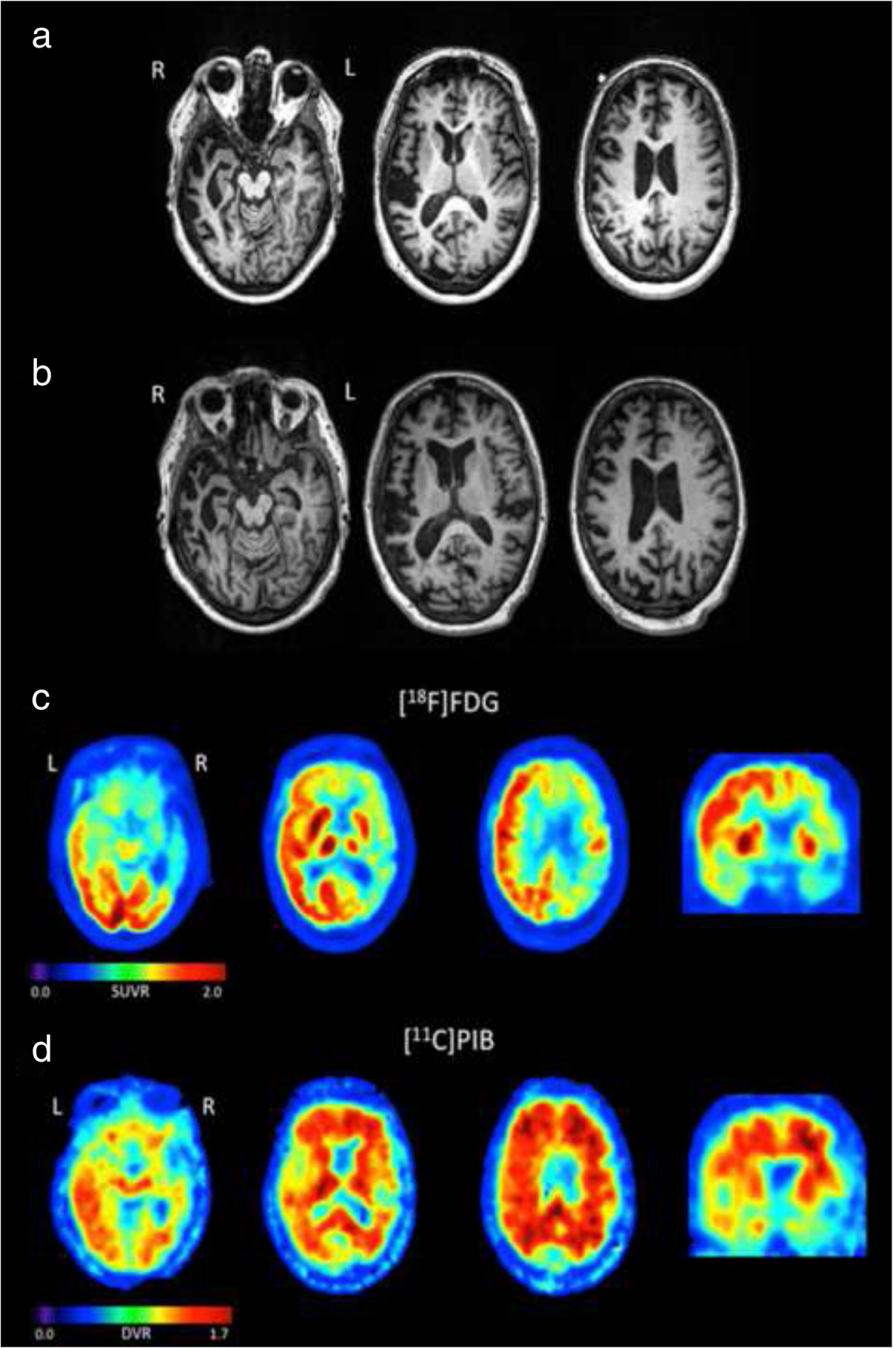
Magnetic resonance imaging
